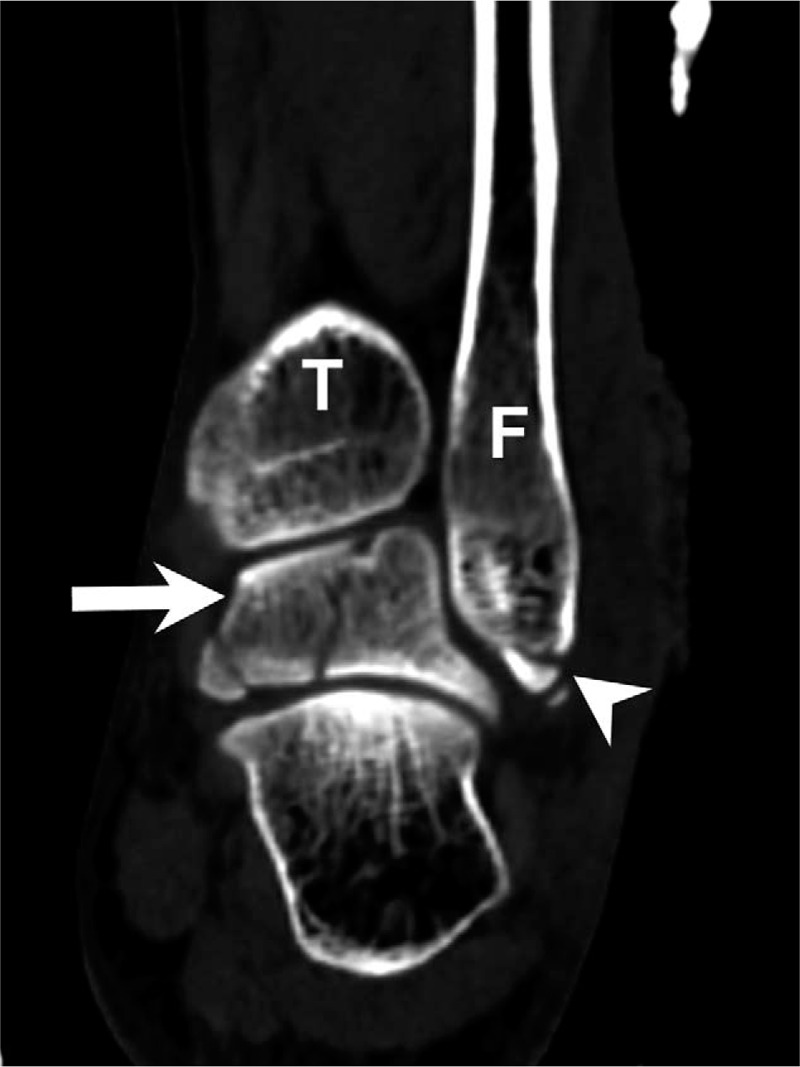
Computed tomography coronary view of the talar fracture with 3 main fragments. Arrowhead = lateral malleolar fracture site, Arrow = talus, F = fibula, T = tibia.
